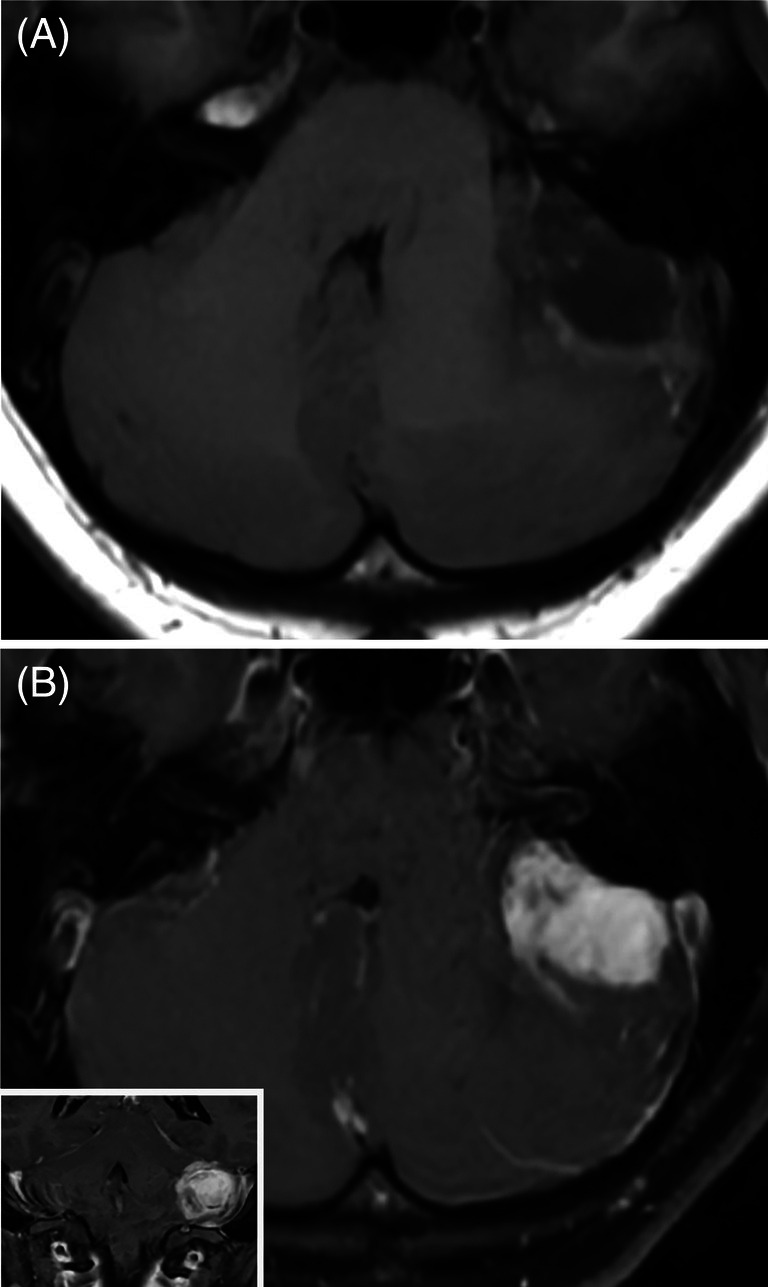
MRI (T1‐FLAIR) pre‐contrast (A) and post‐contrast (B) showed a heterogeneously enhancing mass in the left cerebellar hemisphere.
